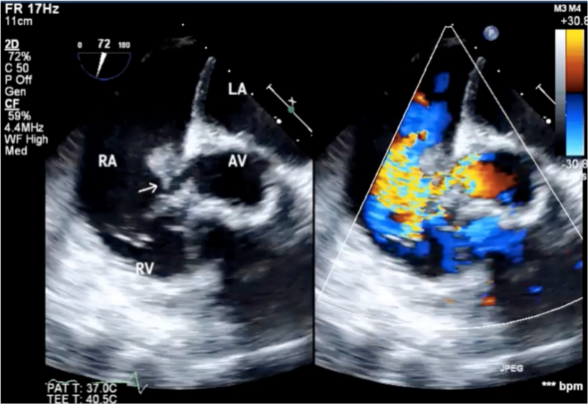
Images of transesophageal echocardiography (TEE) in the mid-esophageal modified aortic valve short axis view. *Left* two-dimensional TEE, demonstrating the vegetation attached to the annulus of the aortic valve (*arrow*). *Right* color Doppler TEE, demonstrating the abnormal flow into the right atrium through the subaortic valve
